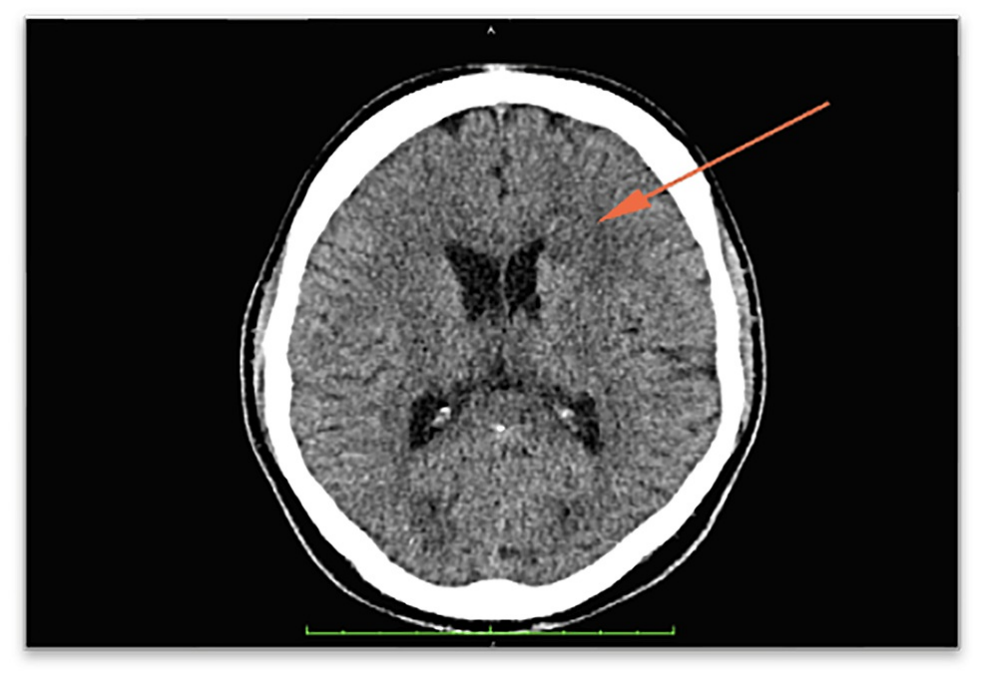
Non-enhanced CT brain showed an ill-defined hypodensity over the left frontal white matter (arrow).
